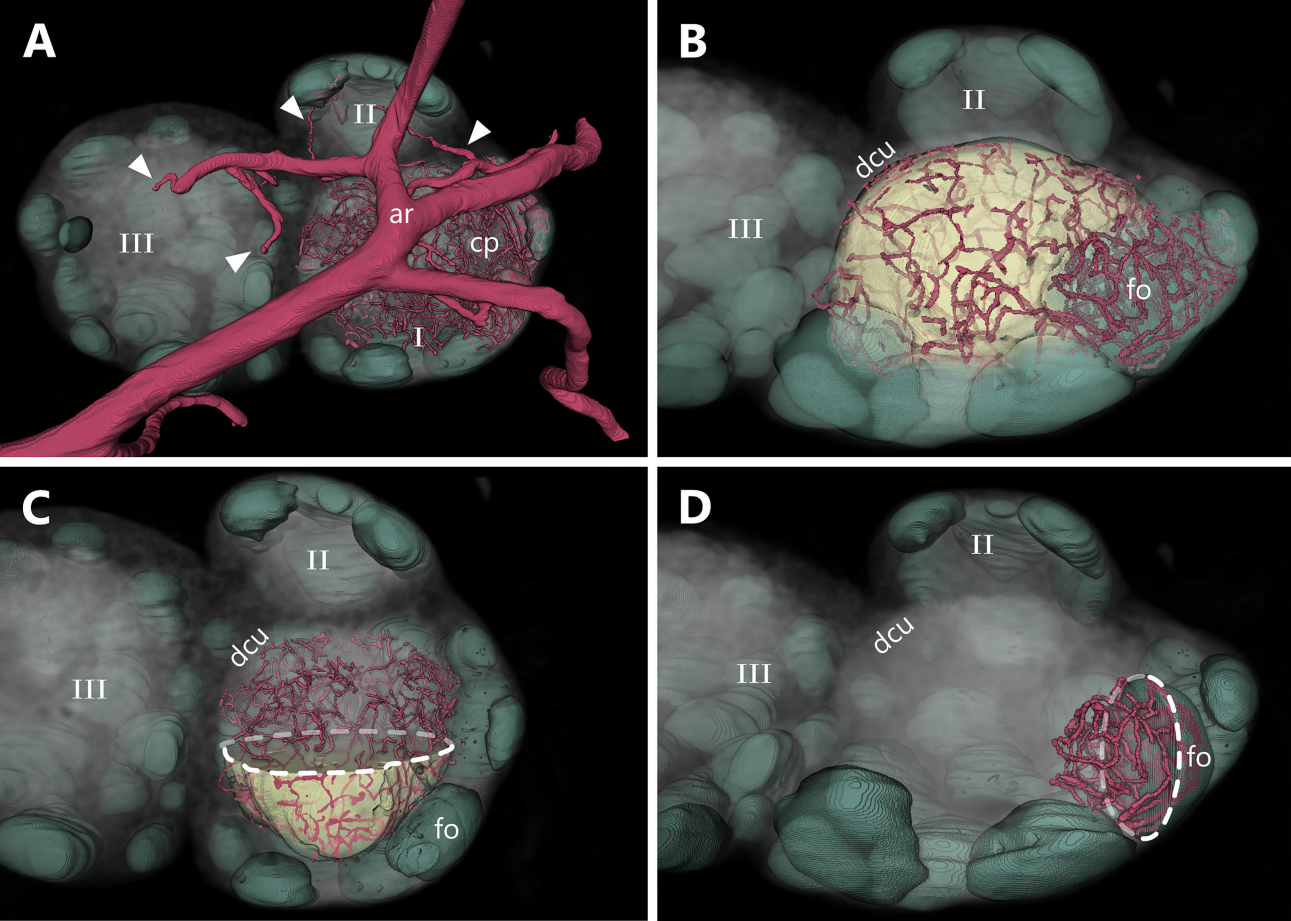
Capillary density between different lymph node (LN) compartments. An animated visualization of this figure is available in the supplementary files (Supplement Video S6). (A-D) Volume rendering of an image stack acquired by means of X-ray phase-contrast imaging with a 2D Talbot array. The LN is displayed in a top view. (A) Overview of the arterial supply of lobe (I) The main arterial trunk feeds multiple arterioles (arrowheads) which further branch into capillary beds throughout the LN. (B) Comparison of capillary density between the deep cortical unit (yellow) and the follicles (mint green) inside lobe (I) For better visualization, the main arterial trunk and the corresponding arterioles are not displayed. (C) To achieve better visualization of capillaries inside the deep cortical unit, the upper part of the reconstructed material is blanked out (dashed line). (D) To achieve better visualization of capillaries inside the B-cell follicle, the left part of the reconstructed follicle material is clipped (dashed line) as well, allowing a better view on the capillary bed inside the follicle. In comparison to the deep cortical unit (panel C), the follicular capillarization appears to be denser. Arterial system=ar (red), capillary system=cp (red), deep cortical unit=dcu (yellow), B-cell follicles=fo (mint green).
